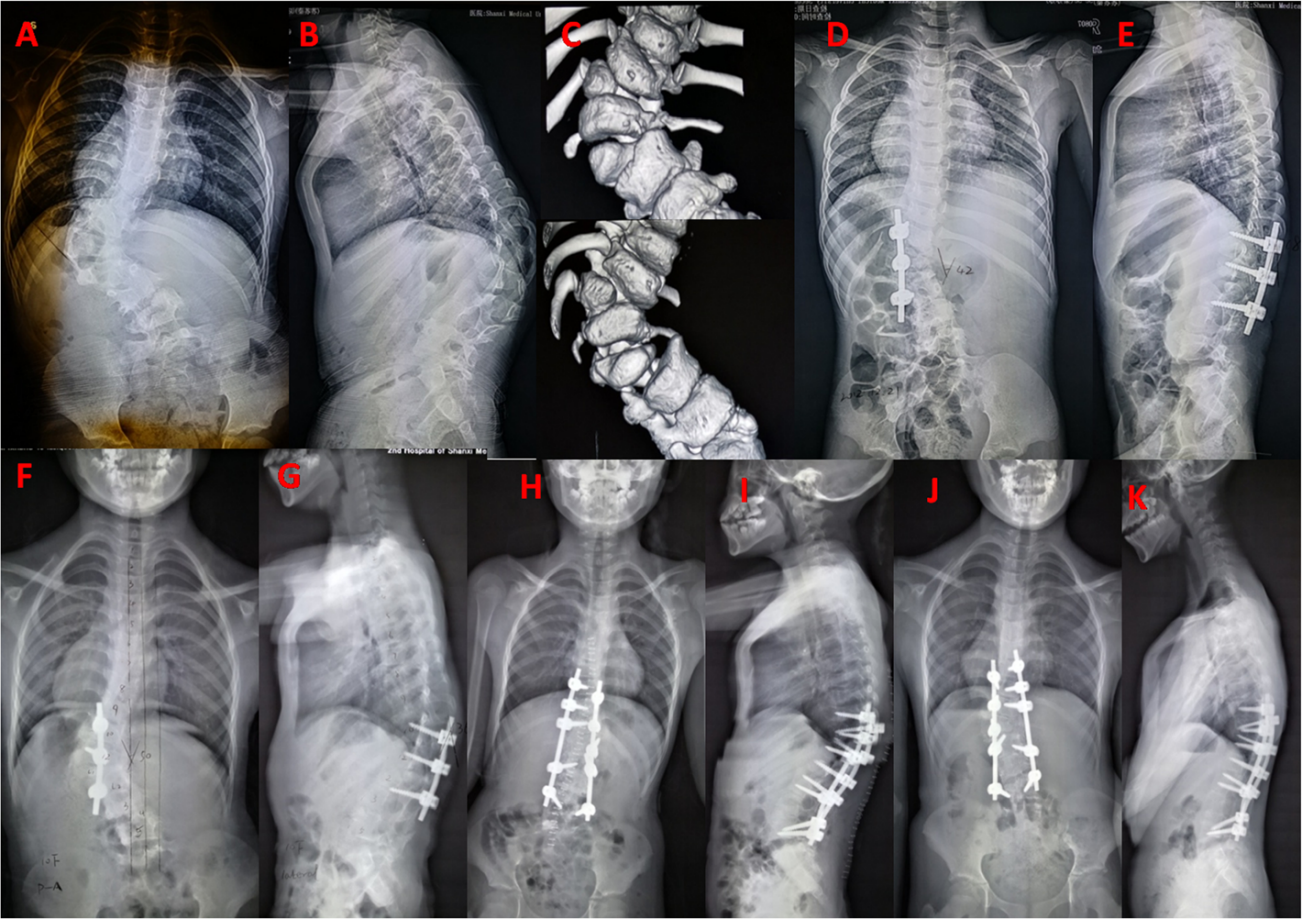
A 7 years old girl with T11, L1 and L4 HV. Radiographs images and 3D-computed tomography images were obtained preoperatively, which shows thoracolumbar kyphoscoliosis due to T11 and L1 full segmentation HV, L4 Semi-segmentation HV (a, b, c). Postoperative PA and lateral radiograph, she was treated with posterior L11,L1 HV excisions with convex fusion (d,e). Curve progression, PJK and L2 pedicle screw plow were presented in 38 months after surgery (f,g). Revision surgery including further hemivertebretomy and extended fixation was performed (h,i). Final PA and lateral radiograph at 67 months follow-up show that correction and trunk balance are well maintained (j,k)
